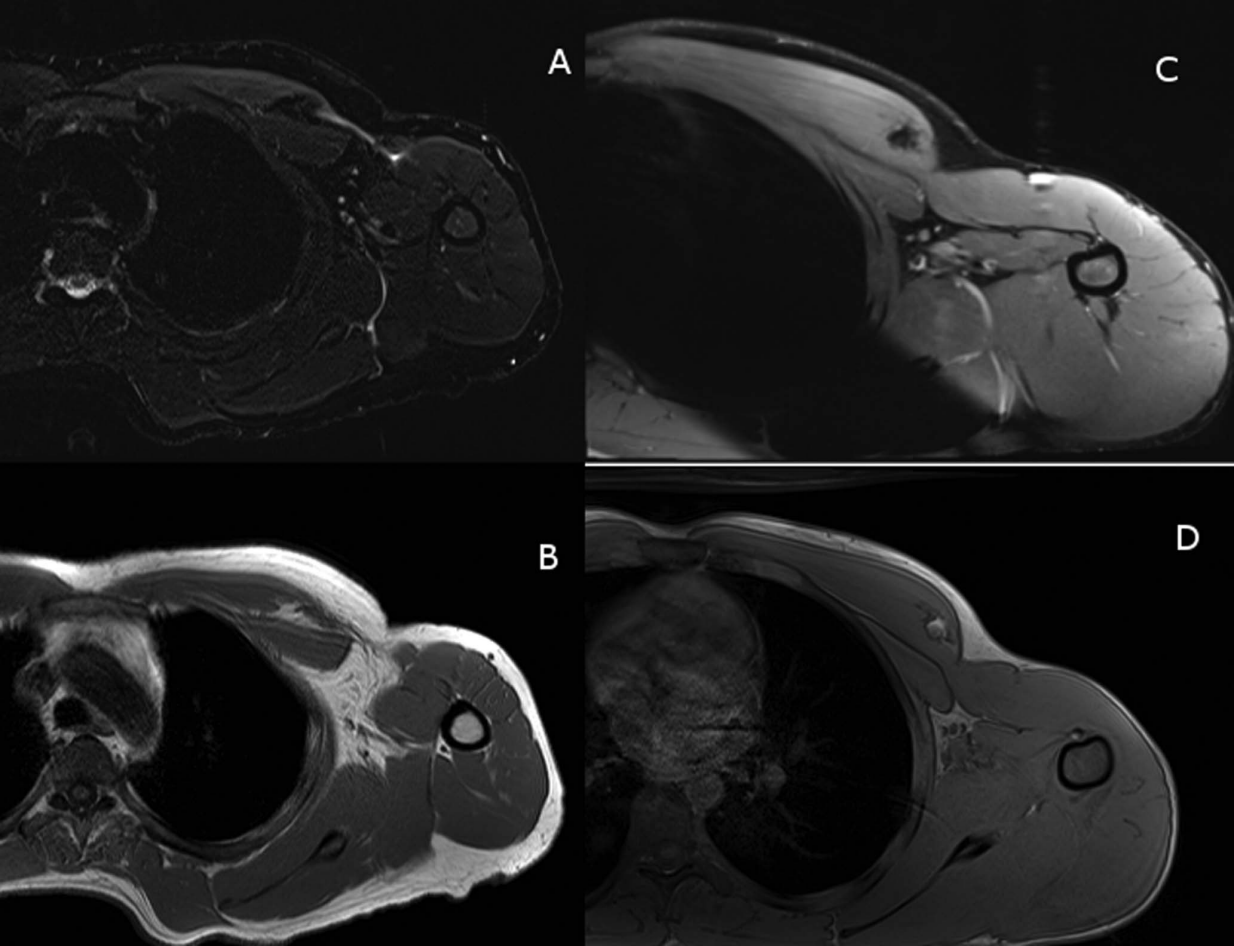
Preoperative magnetic resonance images. (A) Patient 1 axial proton-density-fat-saturation (PD-FS) image demonstrates pectoralis major tendon (PMT) rupture with retraction and a large fluid signal defect. (B) Patient 1 axial T1 image demonstrates tendon retraction and fat-filled defect in the deltopectoral groove. (C) Patient 2 axial PD-FS image demonstrates PMT rupture with marked retraction and bunching. (D) Patient 2 axial T1 image also demonstrates the rupture and confirms a small amount of peritendinous fat retracted with the tendon into the medially displaced myotendinous junction.
